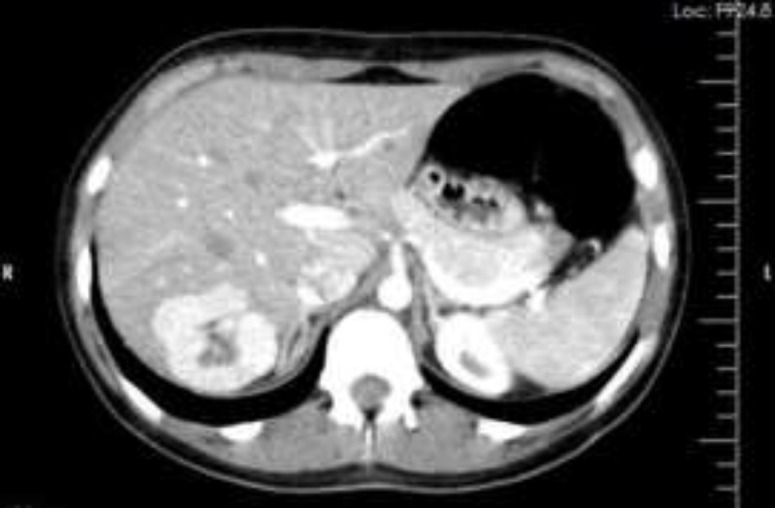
Enhanced axial CT scan through the liver in the arterial phase in a 14-year-old child. The mass demonstrates intense enhancement with a central scar. Solid mass lobulated contour, intense enhancement with a hypodense central area, which is possibly indicative of central scar
